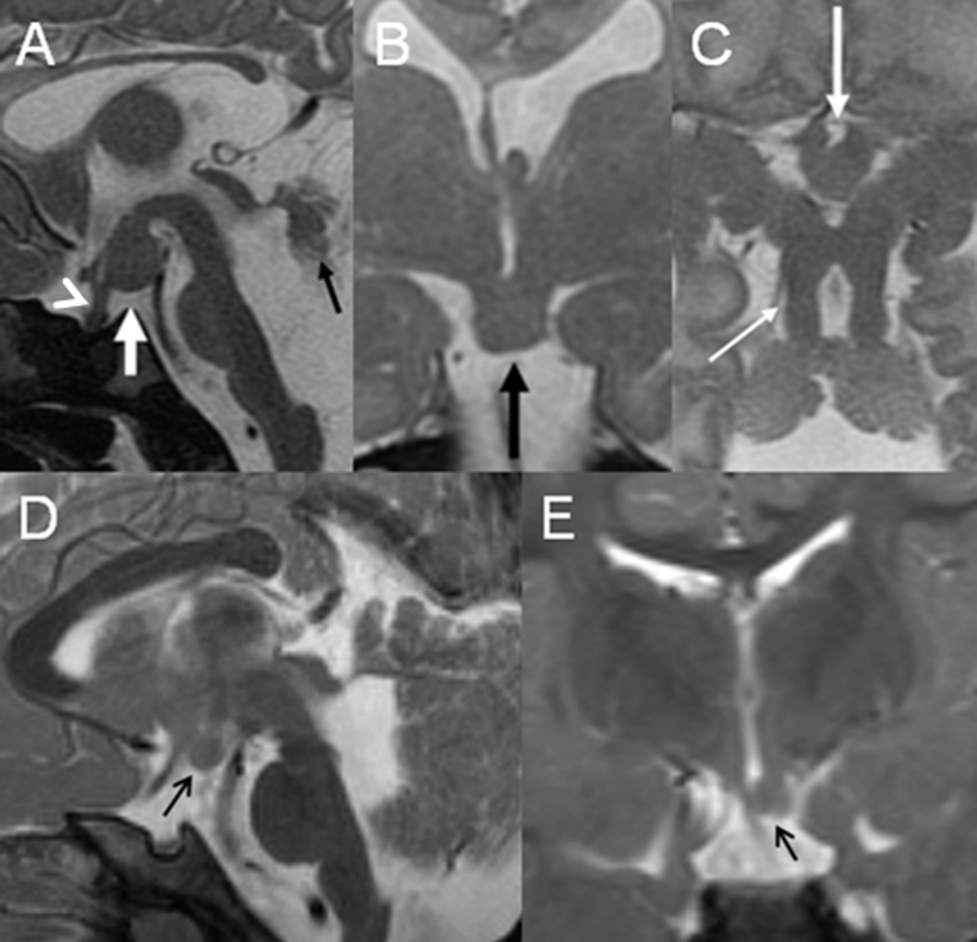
A, midsagittal, B, coronal, and C, axial T2-weighted MR image of a 2-day-old neonate with OFD VI show a hypothalamic hamartoma (white arrow in A, black arrow in B, and thick white arrow in C). Also shown are significant vermian hypoplasia and dysplasia (black arrow in A), enlarged fourth ventricle and posterior fossa, the characteristic MTS (thin white arrow in C), elongation of the mesencephalon, reduced size of the pons, thin corpus callosum, absent of the left leaf of the septum pellucidum, and a thickened pituitary stalk (white arrow head in A; modified from Poretti A et al, AJNR, 2008, with permission). D, midsagittal and E, coronal T2-weighted MR image of a 2.3-year-old boy with OFD VI show a left paramedian hypothalamic hamartoma (black arrow). Moreover, D shows hypoplasia and dysplasia of the cerebellar vermis and enlargement of the fourth ventricle.
